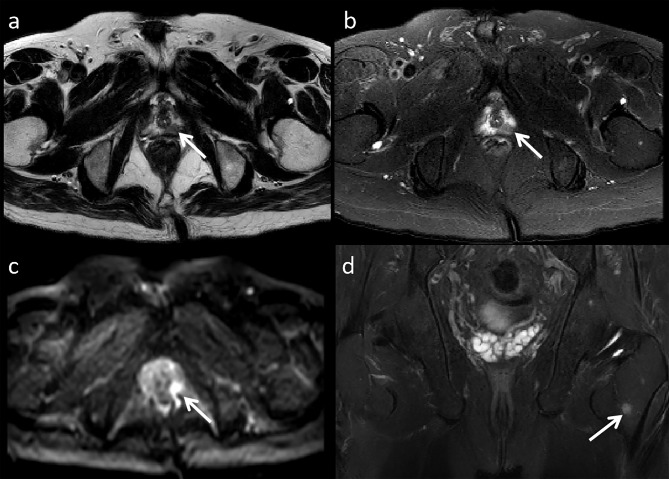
MRI examination of the pelvic cavity. a T2WI showed a hypointense nodule in the left peripheral zone of the prostate. b Axial fat suppressed T2-WI showed a low signal nodule in the prostate. c High b-value DWI map showed a focal area of diffusion restriction in the the prostate. d Coronal fat suppressed T2-WI showed high signal in left upper femur
